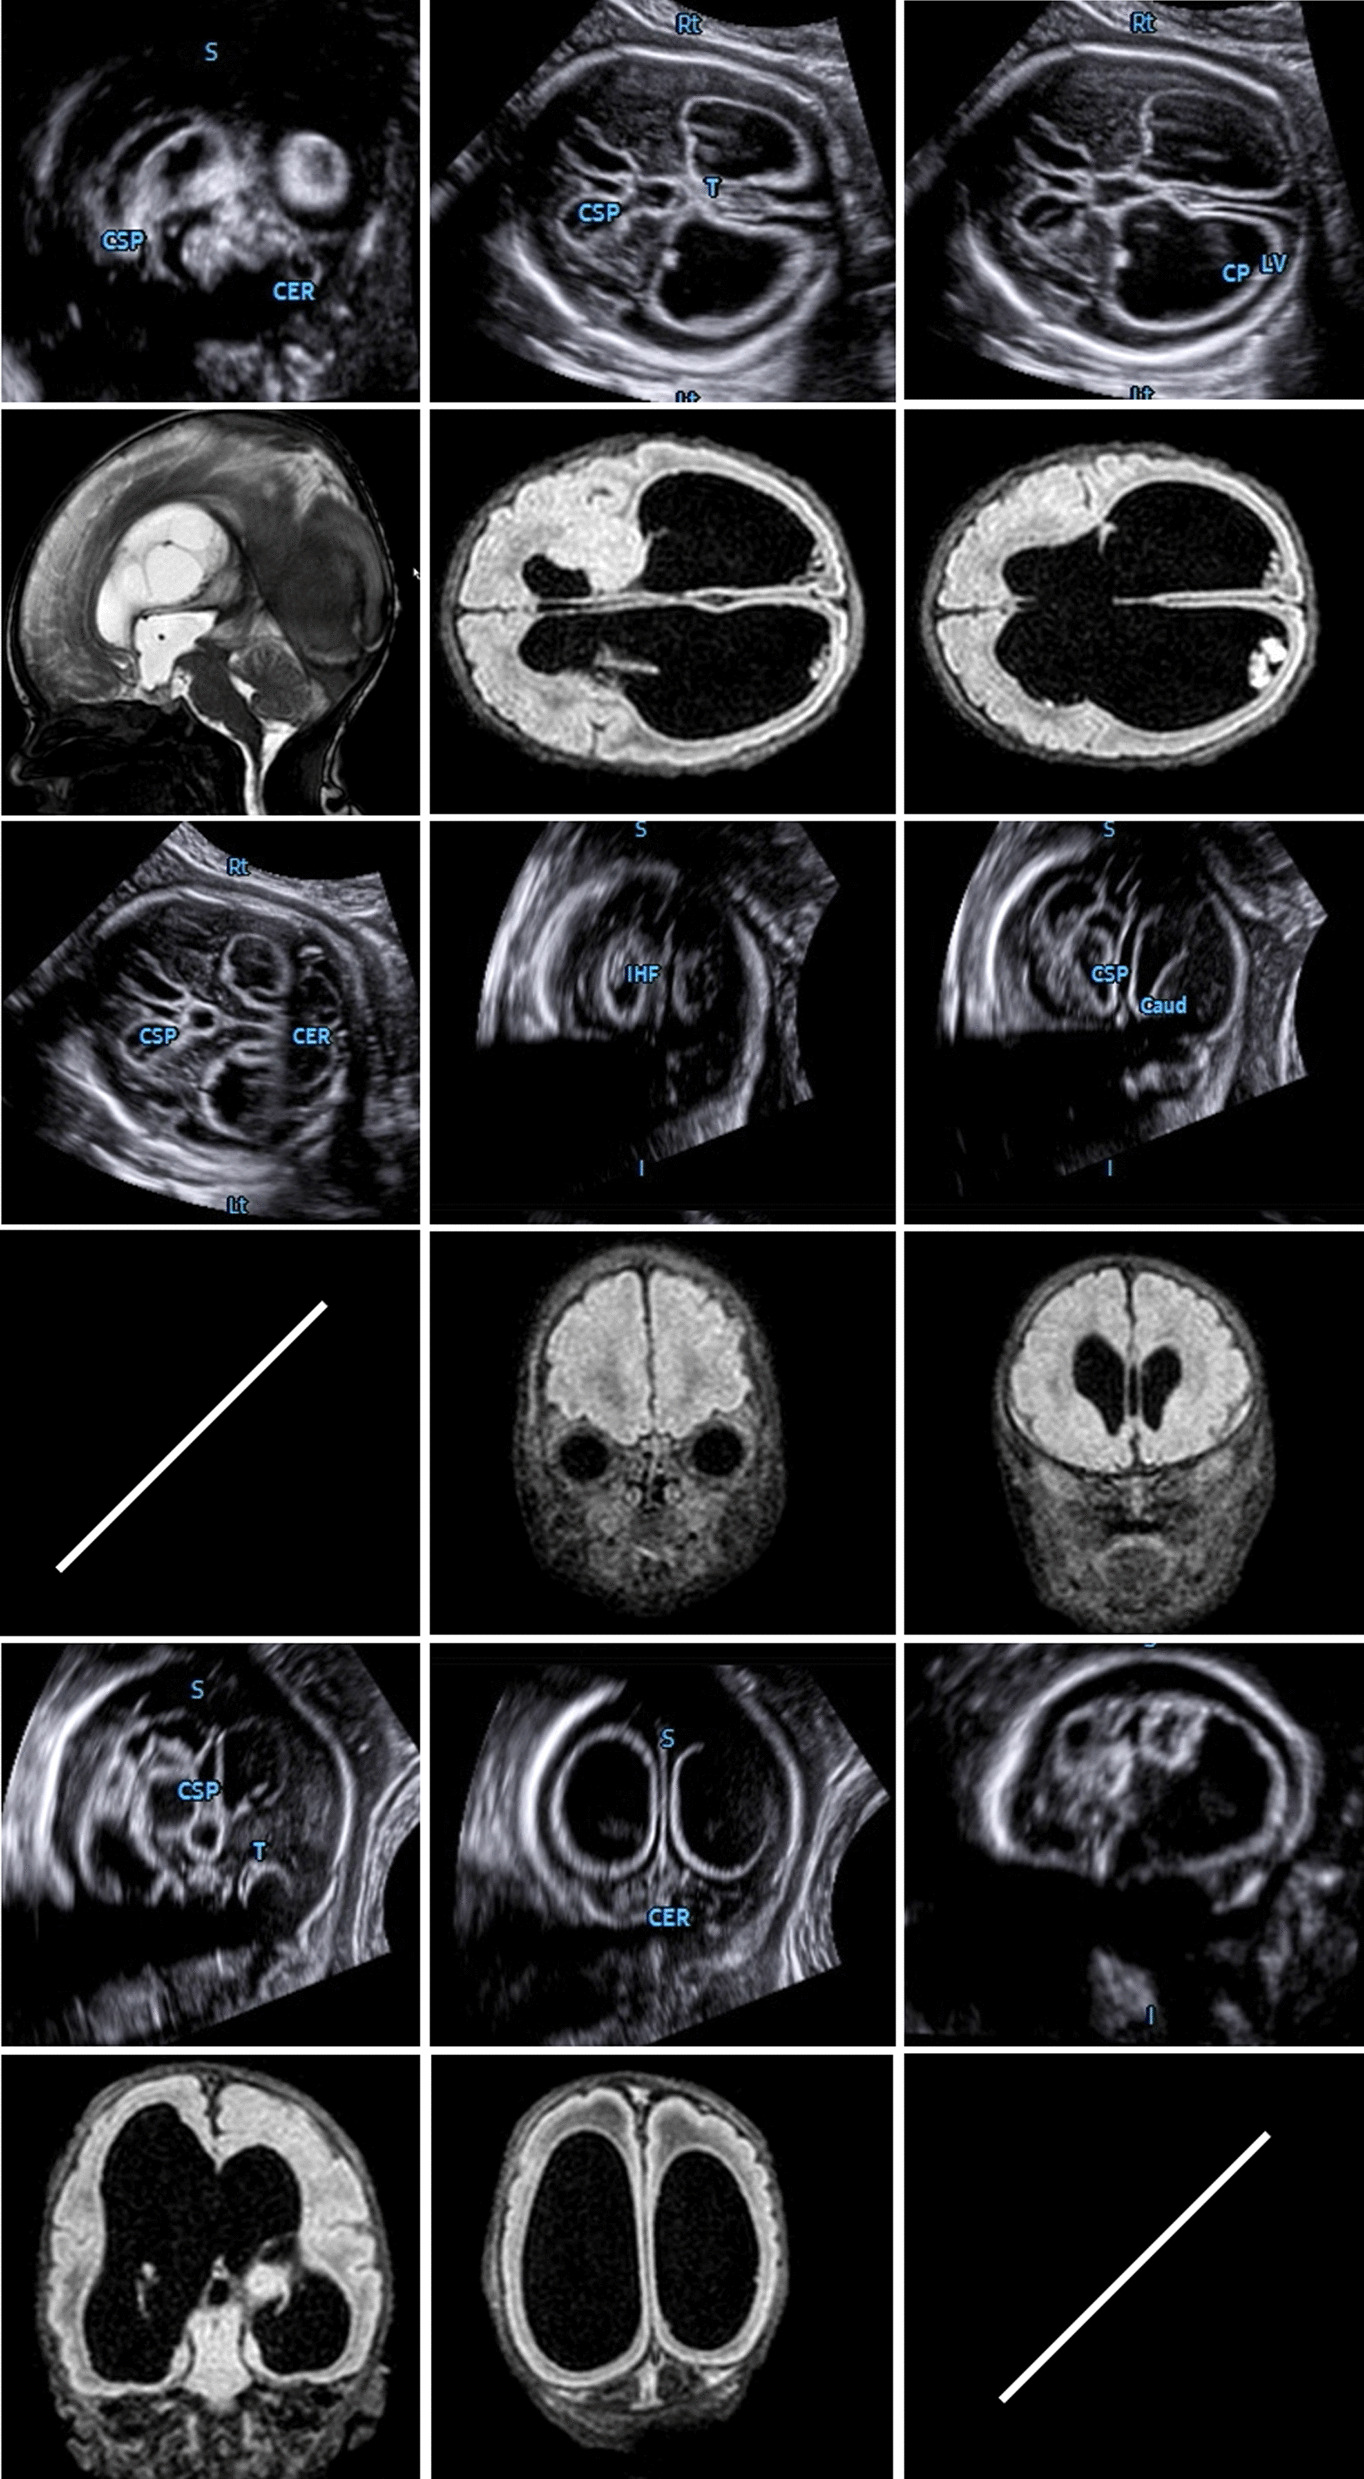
Intraventricular hemorrhage delineated using 5D CNS+™ in utero at 35 gestational weeks and correlated to the corresponding MR appearance on the 1st day after cesarean section. Note the severe hydrocephalus and the echogenic outlining of the ventricles. The blood clots predominantly seen in the axial and anterior coronal planes during prenatal imaging were markedly degraded in size or could not be reproduced postnatally. However, severe asymmetric enlargement of the ventricular system remained, and the cerebral cortex was thinned, corroborating the prenatal findings. MR images that correspond exactly for both the transcerebellar and parasagittal planes from 5D CNS+™ reconstruction could not be assigned due to the predetermined oblique cutting section.
